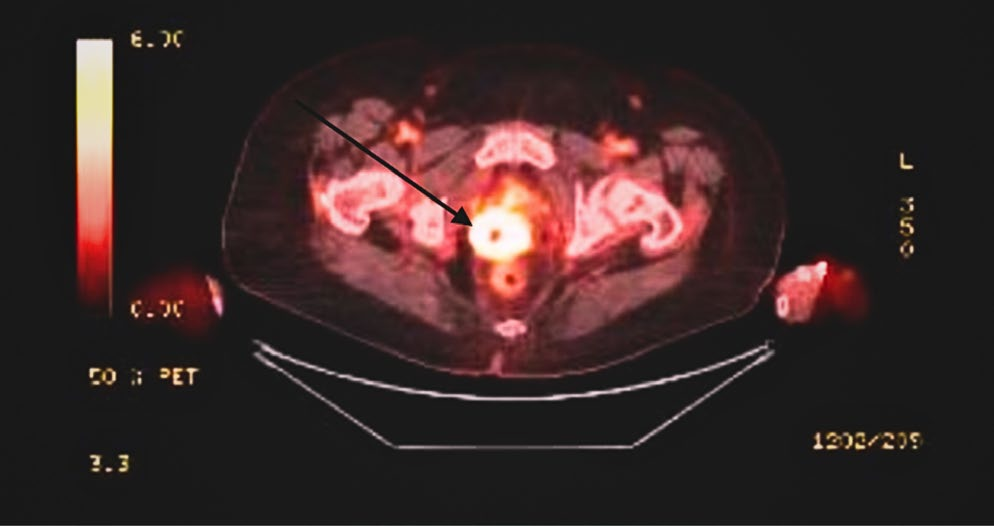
FDG‐PET/CT Imaging of Vaginal Cuff Lesion. The figure displays an axial FDG‐PET/CT image showing an intensely FDG‐avid lesion with a photopenic center, corresponding to the vaginal cuff lesion. The maximum standardized uptake value (SUV) of the lesion is 17.6, indicating significant metabolic activity. The findings suggest biologic tumor activity at this site.
